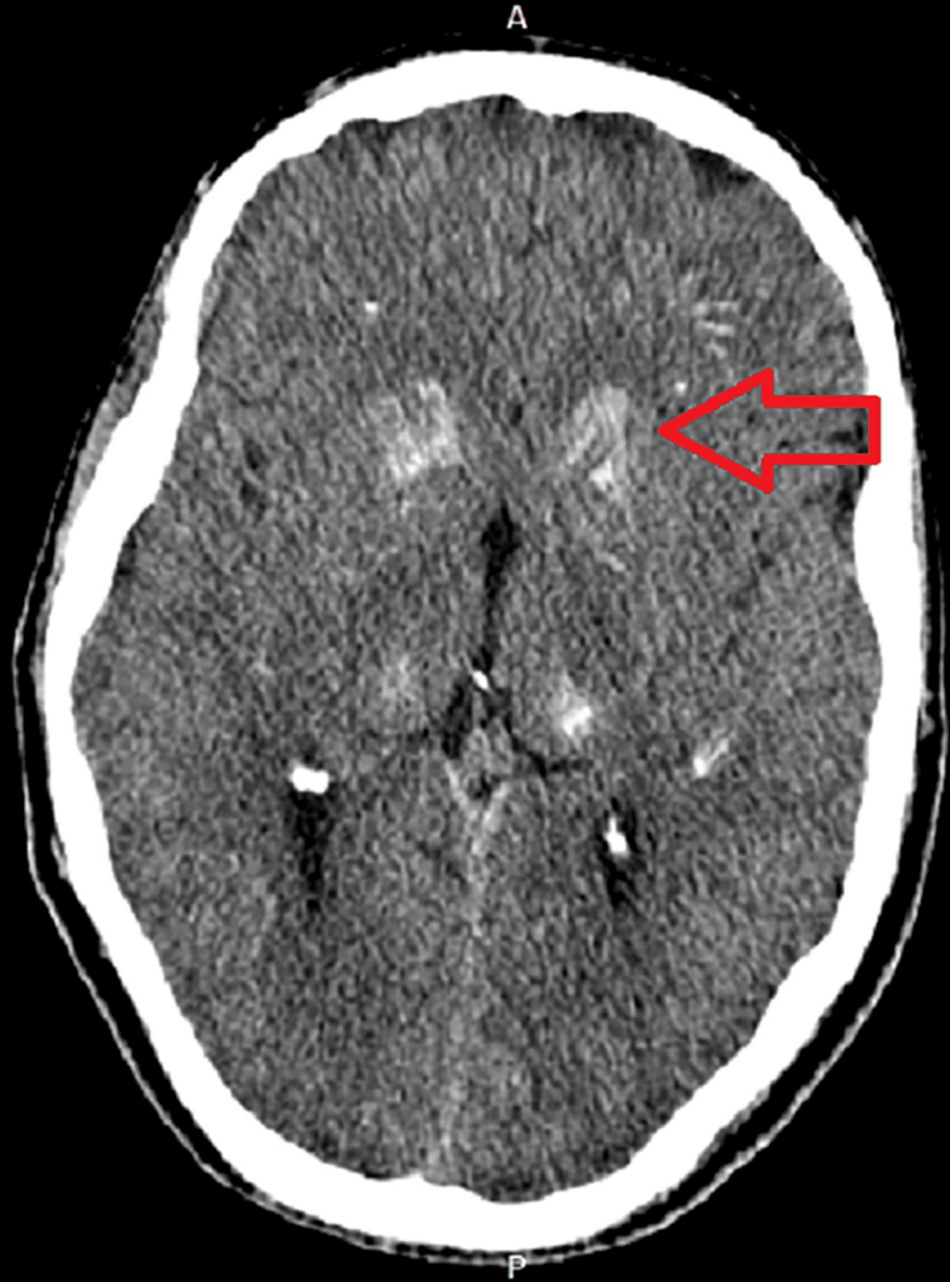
Non-contrast computed tomography image showing bilateral dense calcifications (red arrow).
